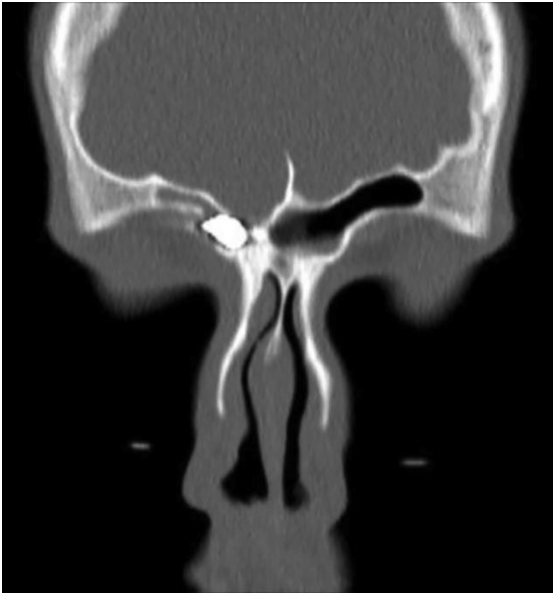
CT revealing a fractured posterior table of the frontal sinus. Coronal CT of the paranasal sinuses on bone window showing a fractured posterior table of the frontal sinus.
